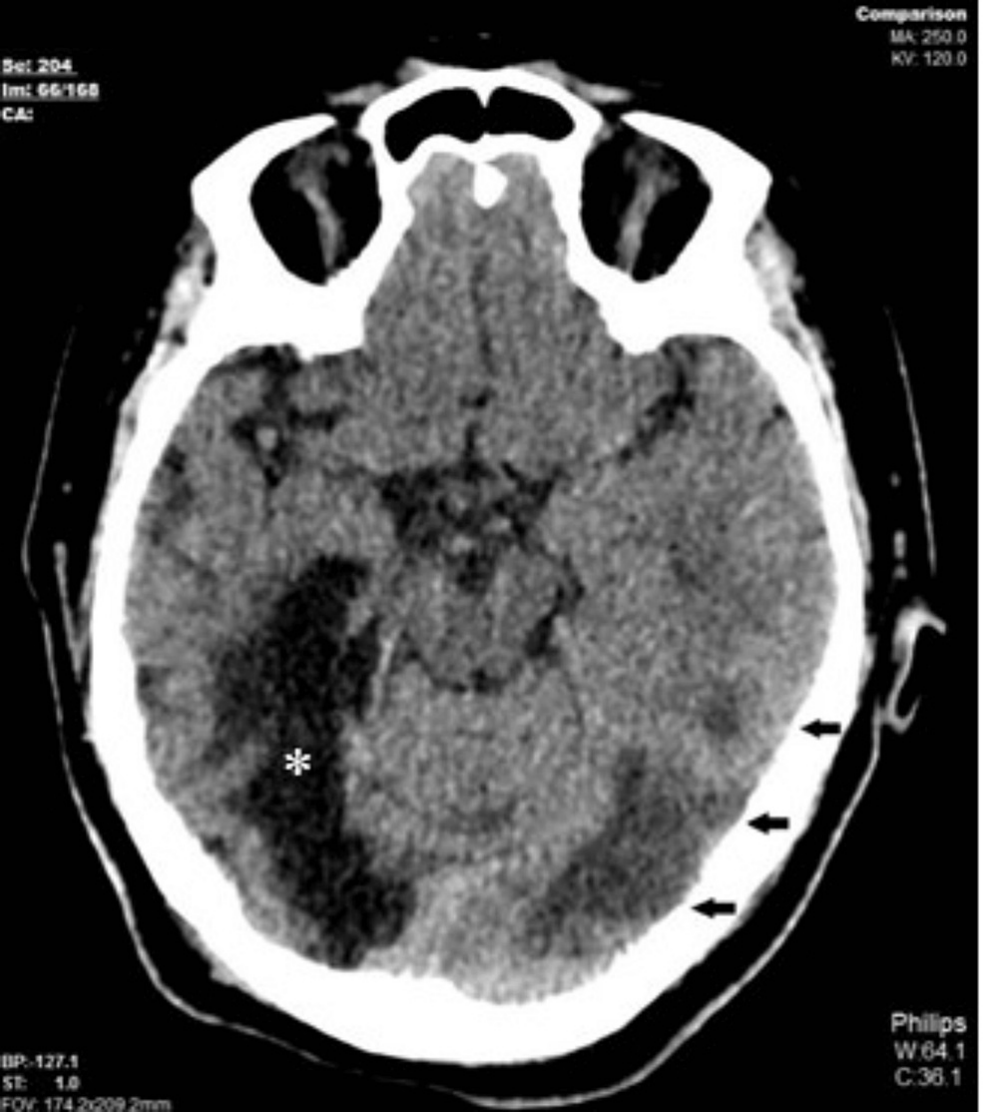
CT of the brain in the axial plane showed ill-defined hypodensities in the left occipital lobe (black arrows), consistent with a recent infarct. Another well-defined hypodensity was noted in the right parieto-occipital-temporal lobe, along with ex vacuo dilatation of the temporal horn of the right lateral ventricle, in keeping with encephalomalacic changes due to a previous right PCA territory infarct (*). CT: computed tomography; PCA: posterior cerebral artery.
